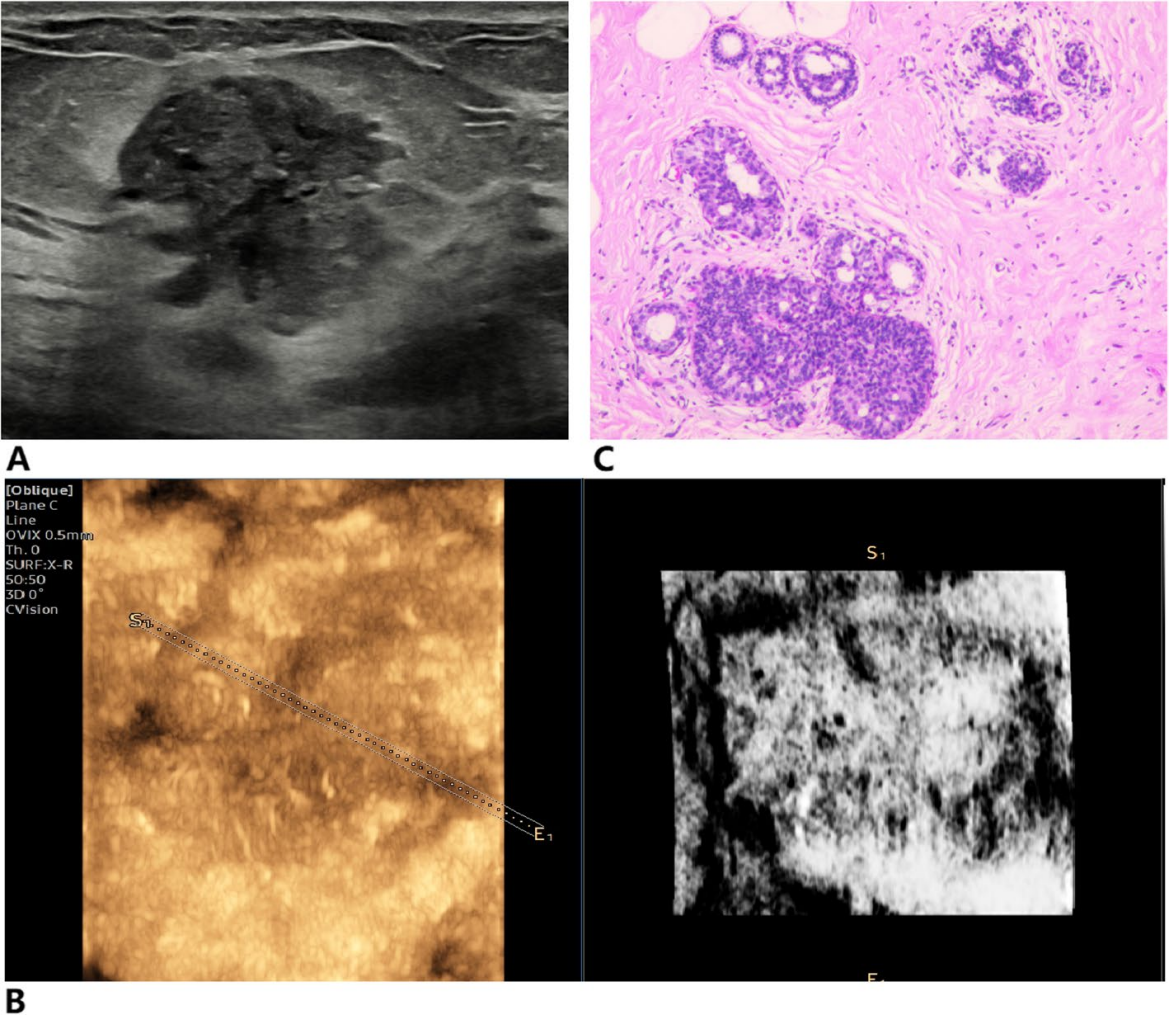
Mammary adenosis of the left breast. A BI-RADS 4B breast mass in ultrasound image. B Inversion imaging showing diffuse distribution of NTR within the mass, the inversion score was 1. C Pathological section showing that the mass was a mammary adenosis with a small amount of surrounding duct dilation
